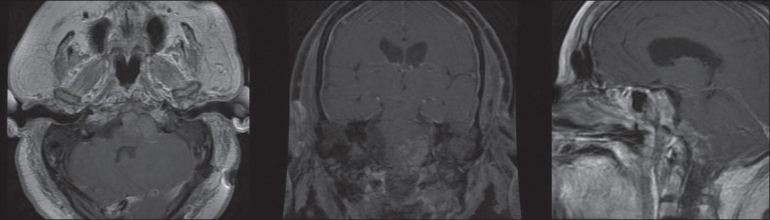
Postoperative MRI of the brain T1 postcontrast infusion sequences in axial, coronal, and sagittal planes demonstrating extent of surgical debulking
